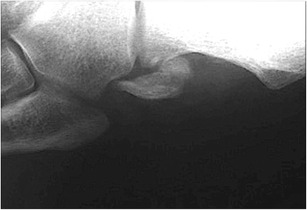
POPS – standard radiograph. Radiographs can show an enlarged and sclerotic OP suggestive of a stress fracture
